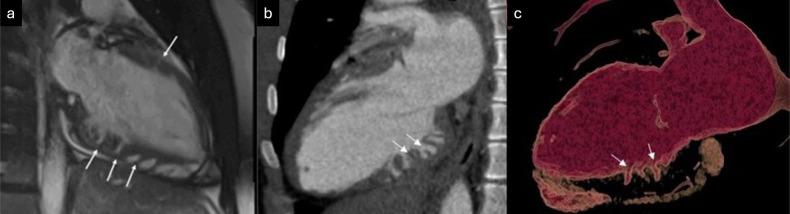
Myocardial invaginations perpendicular to the endomyocardial surface, predominantly in the inferoseptal wall of the LV, corresponding to myocardial crypts (arrows). These can be identified both on CMR (a SSFP cine two-chamber view) and CT (b two-chamber multiplanar reconstruction, c two-chamber volume rendering)
